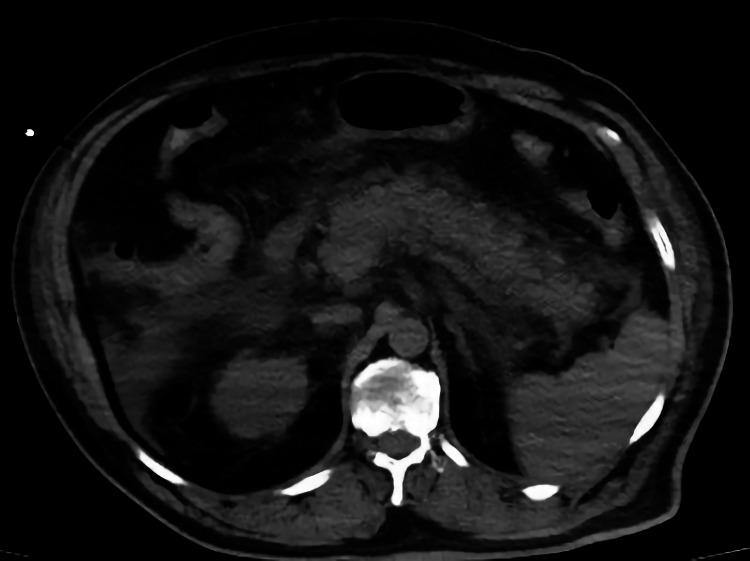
Increase in the size of the pancreas Non-contrast axial CT image showing an increase in pancreas' size CT: computed tomography
